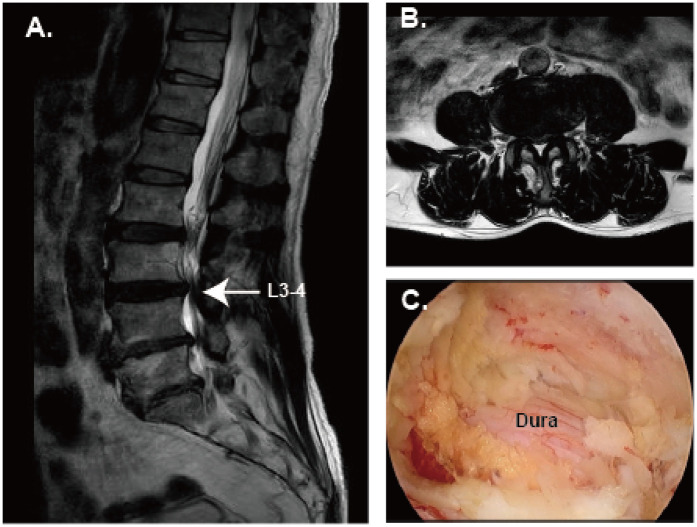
Preoperative MRI and intraoperative UBE images of the patient. Preoperative MRI indicated lumbar spinal canal stenosis at the L3-4 level (A,B). Intraoperative visualization UBE confirmed adequate decompression of the thecal sac (C).
